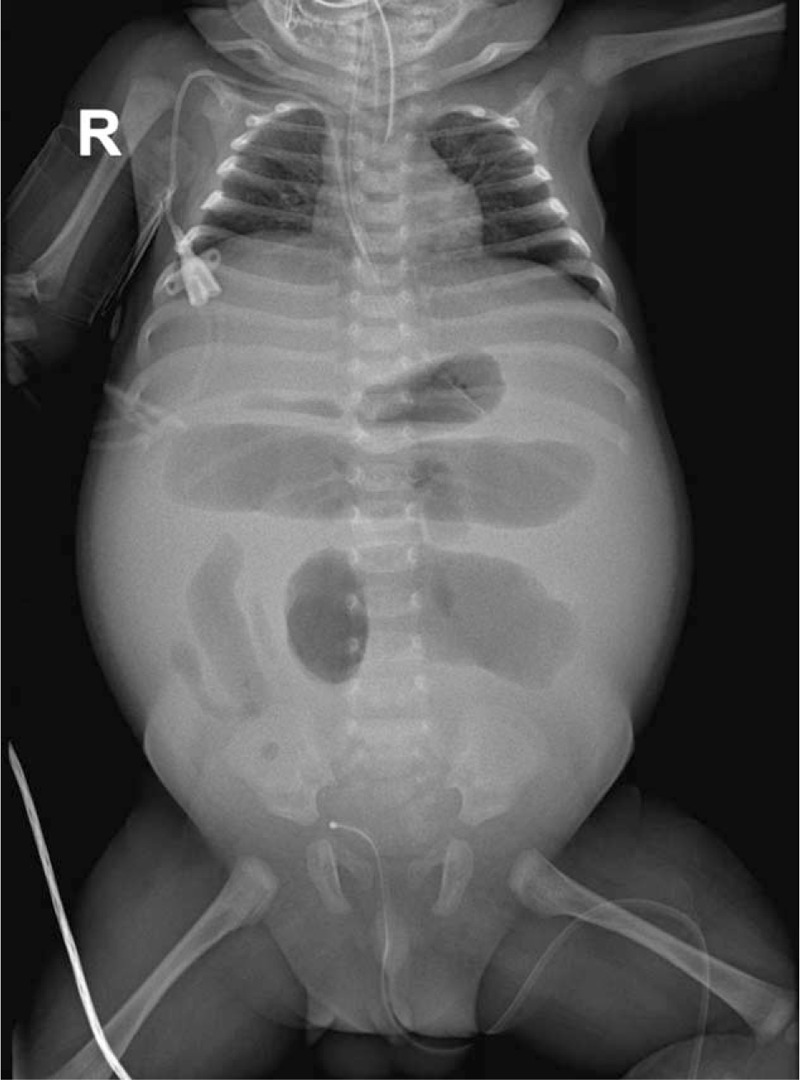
Initial plain radiography on PICU admission. Abdomen was distended with colon wall dilatation to approximately 5 cm with bowel edema.
